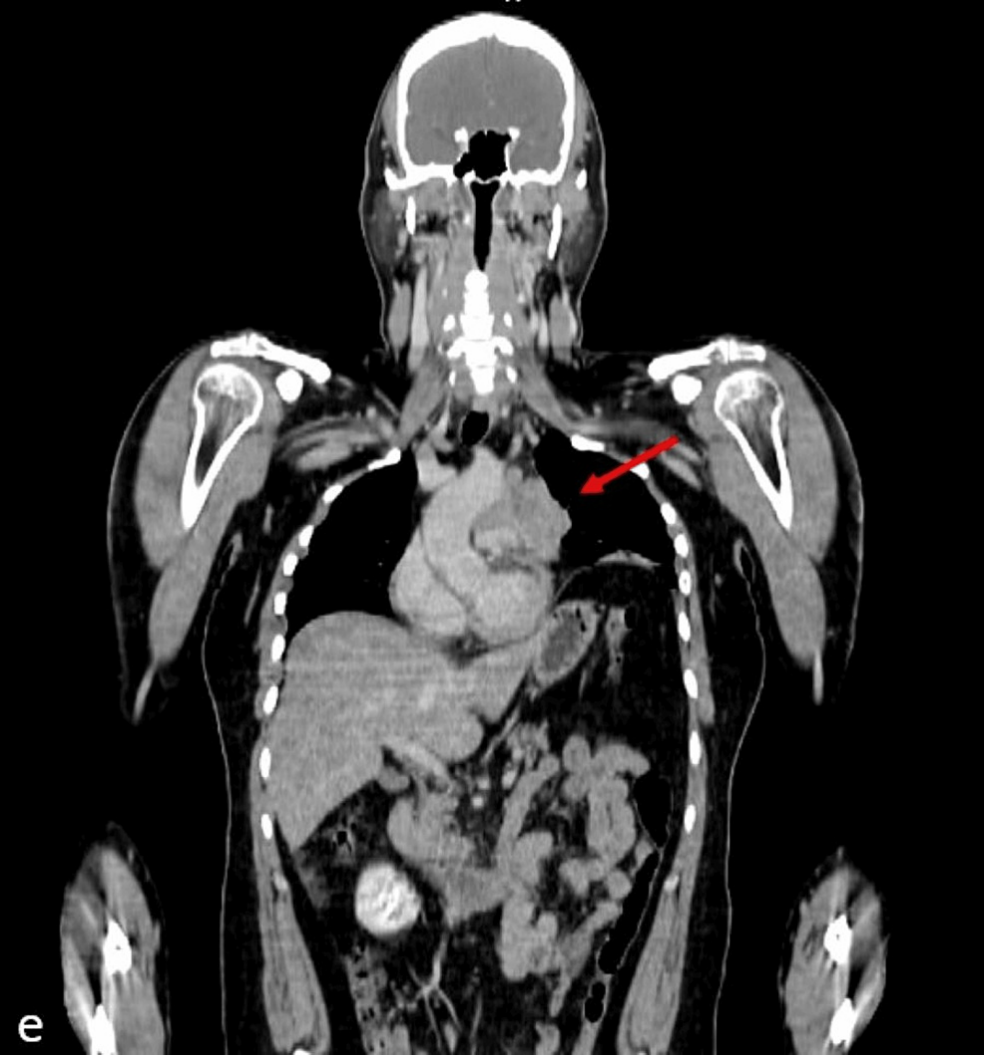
Coronal contrast-enhanced computed tomography (CECT) image of the chest and abdomen
